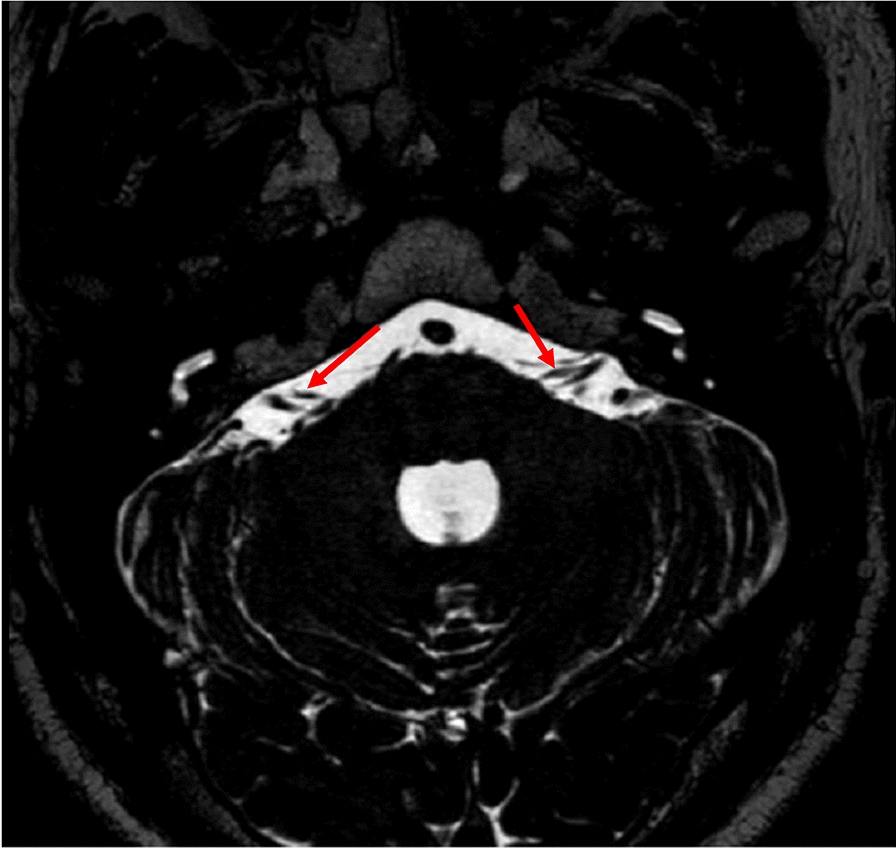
Normal MRI anatomy of cisternal segment of the facial nerve: axial T2-weighed MRI image showing the cisternal part of the facial nerve with the red arrows, anterior to CN VIII
